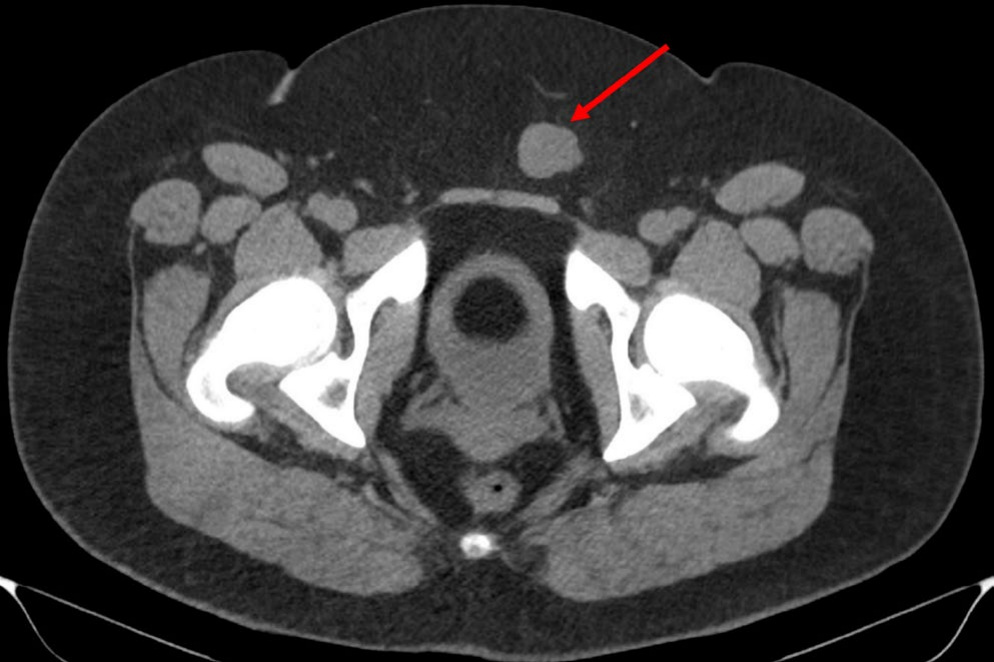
CT abdomen and pelvis without IV contrast performed 5 months prior to the patient's diagnosis reveals a round mass in the subcutaneous tissues anterior to the pubic symphysis that is isodense to muscle (red arrow). At this time, the mass measured 2.0 × 2.7 cm (AP × TR) with no significant surrounding edema.
